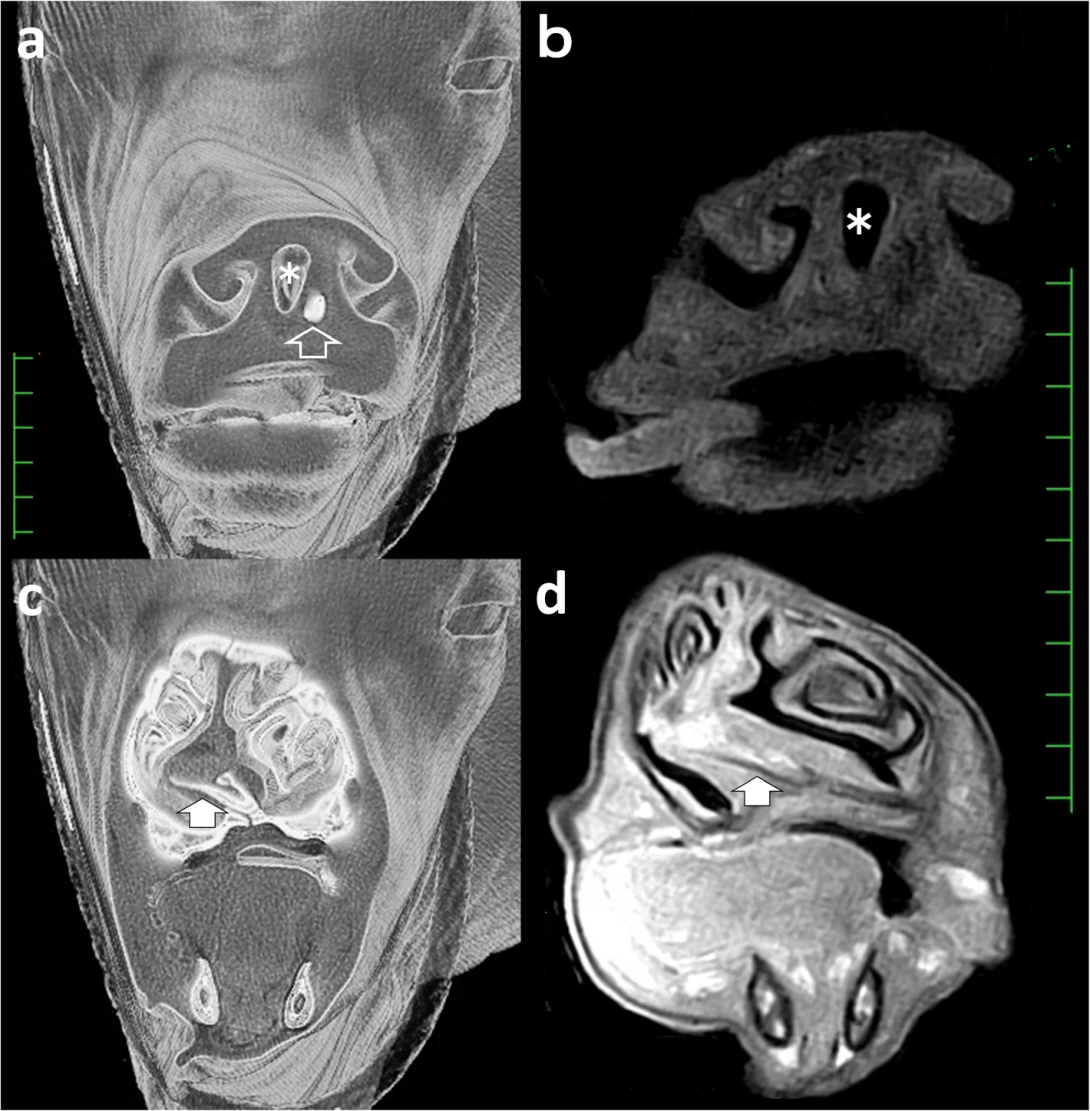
Transverse 3D-CT and T1-weighted MRI corresponding to approximately 1 cm (a and b) and 7 cm (c and d) deeper than the muzzle. a A bone-like structure (empty arrow) is observed in the left inferior site of the middle nostril (asterisk). b An elliptical middle nostril (asterisk) is seen in the space between the right and left nostrils. c The hyperdense vomer (white arrow) leans toward the right, along the transformed nasal septum. d The transformed vomer (white arrow) is imaged as a low-signal-intensity structure. The transformed turbinate bones are also clear. Scale: 10 mm on CT and MRI
